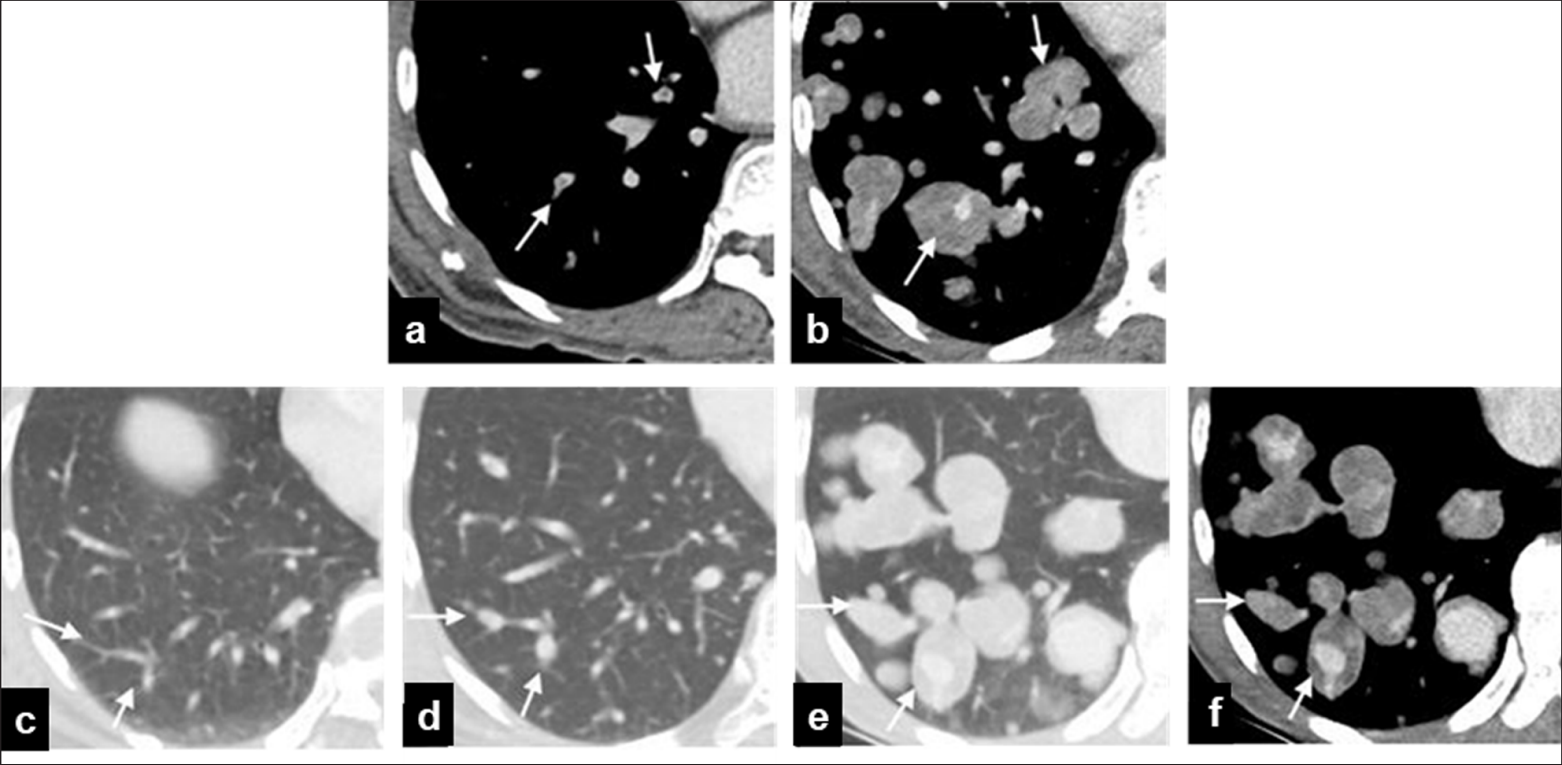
(a) and (b) Serial axial chest CT within one month showing aneurysmal dilatation and developing soft tissue masses surrounding the previously seen tumor emboli in the peripheral branches of right pulmonary arteries (arrows). (c–f) Serial axial chest CT at eight months prior (c), one month prior (d), and the present study (e) and (f) showing gradual dilatation of peripheral branches of right pulmonary arteries, and finally pseudoaneurysms with surrounding soft tissue masses (arrows).
